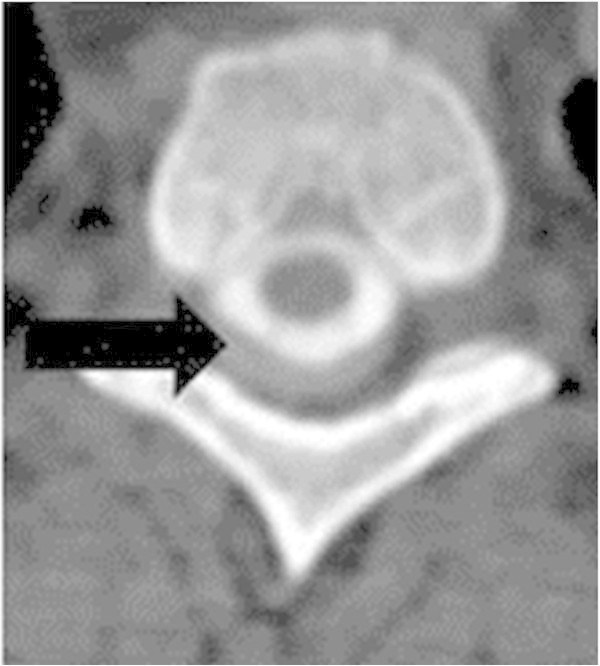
Case I: Axial Myelo- CT demonstrating contrast leakage around the spinal cord.
